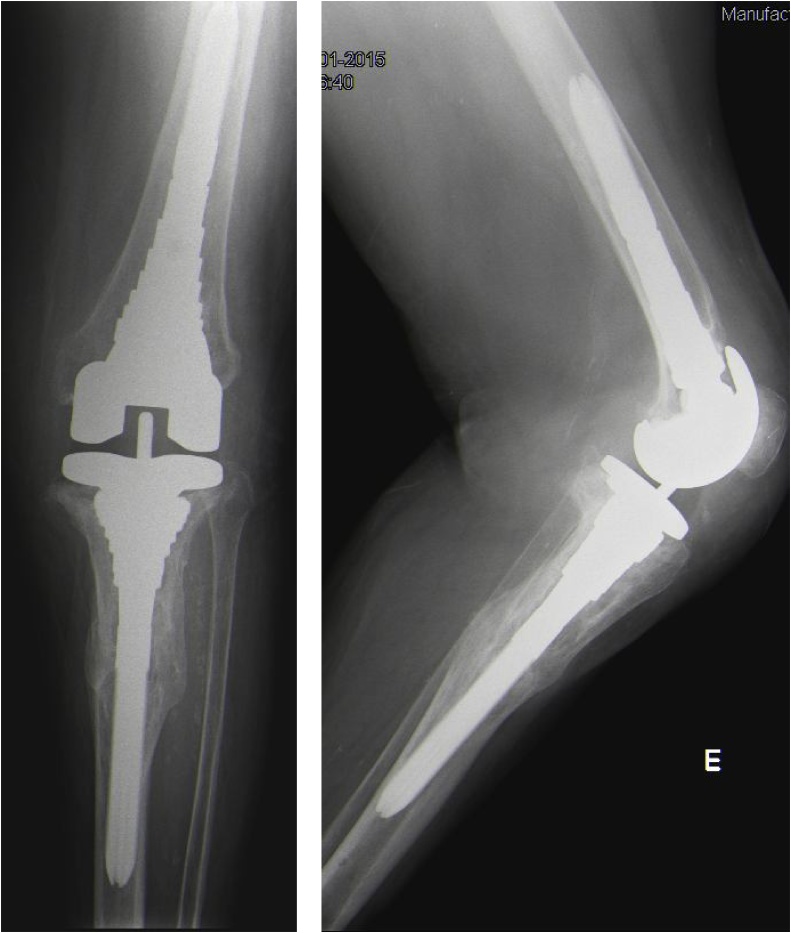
Antero-posterior and lateral x-ray view of the semi-constrained revision total knee arthroplasty with prolonged stems into the femoral and tibial medullary canal.
